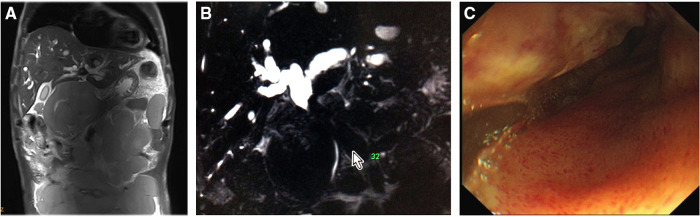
Initial findings. (A) Abdominal magnetic resonance imaging showed massive lymphomatous lesions filling the peritoneal cavity. (B) Magnetic resonance cholangiopancreatography revealed an external compressive stricture in the superior middle common bile duct and dilation of the intrahepatic and extrahepatic ducts. (C) Endoscopic retrograde cholangiopancreatography showed ductal descending-junction stenosis of the duodenal bulb caused by lymphoma infiltration.
